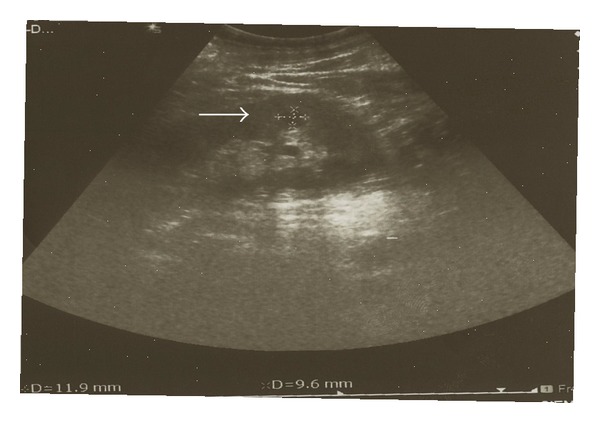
Ultrasound showing the renal abscess which appears as a hypoechoic area 1.19 × 0.96 cm within the cortex of the left kidney.
